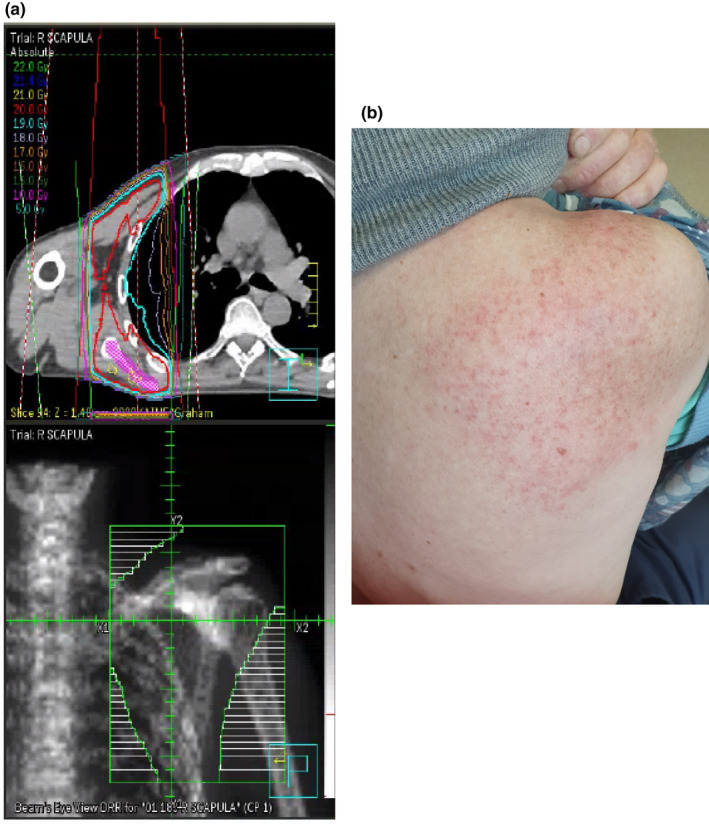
A, (top). Axial computed tomographic image of radiation distribution for right scapular radiotherapy. The target is outlined in pink. A, (bottom). Digitally reconstructed radiograph showing scapular irradiation field. Horizontal bars indicate shielded areas. B, Cutaneous eruption at the site of previous scapular irradiation
